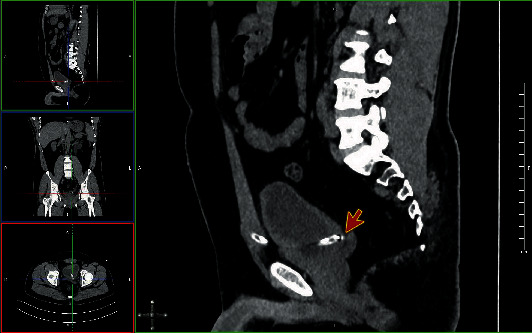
Computed tomography (CT) abdomen, sagittal view showing the tip of the suprapubic catheter inserted at the left ureterovesical junction (red arrow).
